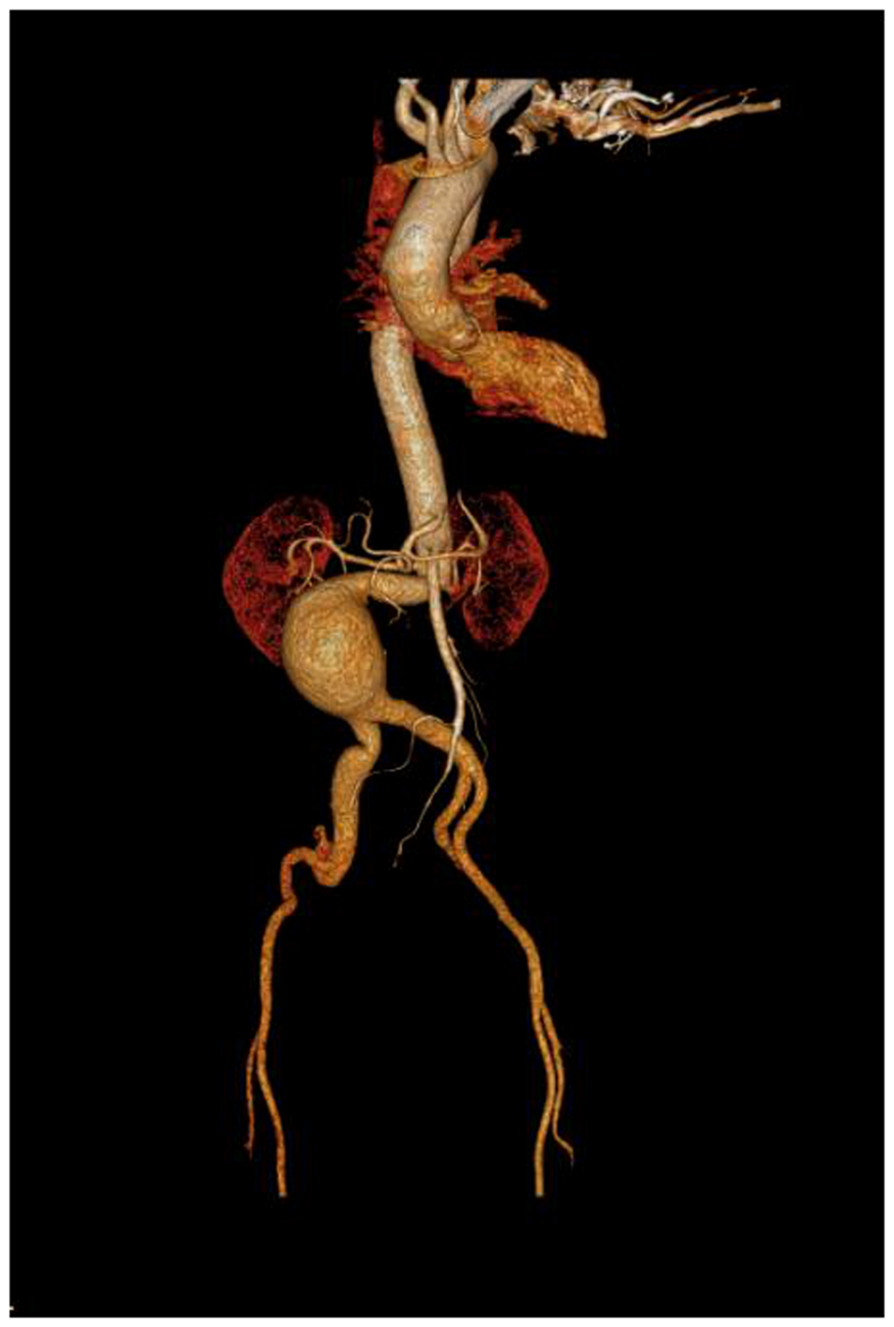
Computed tomography showing an infrarenal AAA with a sharp angled neck and a short neck. The right common iliac artery was severely tortuous.
